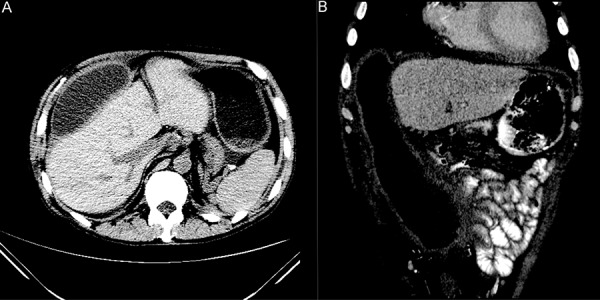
A: Axial CT noncontrasted image at the level of the porta hepatis obtained 18 weeks after index admission. There has been interval development of a loculated, thick walled fluid collection anterior to the liver. The perisplenic and perigastric fluid is no longer present. B: Coronal reconstructed image through the anterior abdomen at 18 weeks demonstrates the craniocaudal extent of the encapsulated fluid collection which extends from the right subphrenic space into the pelvis. Although the collection closely approximates and exerts a mass effect on adjacent small bowel, there is no invasion or trapping of bowel.
